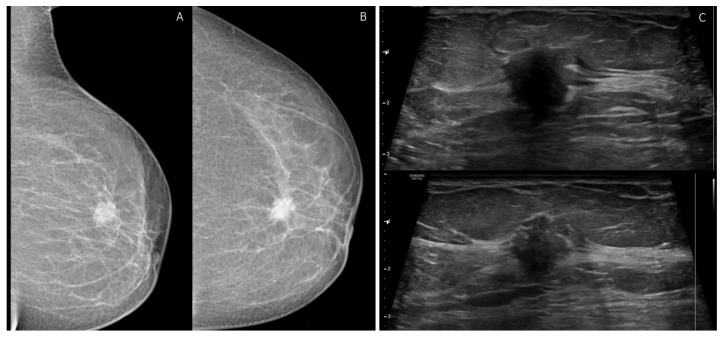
BI-RADS category 4C: 40-year-old woman with invasive ductal carcinoma. (A,B) left mediolateral oblique and craniocaudal digital mammogram show irregular high-density mass with spiculated margins. (C) transverse ultrasound shows an irregular mass with angular margins and posterior shadowing corresponding to mammographic findings. No suspicious axillary LNs.
